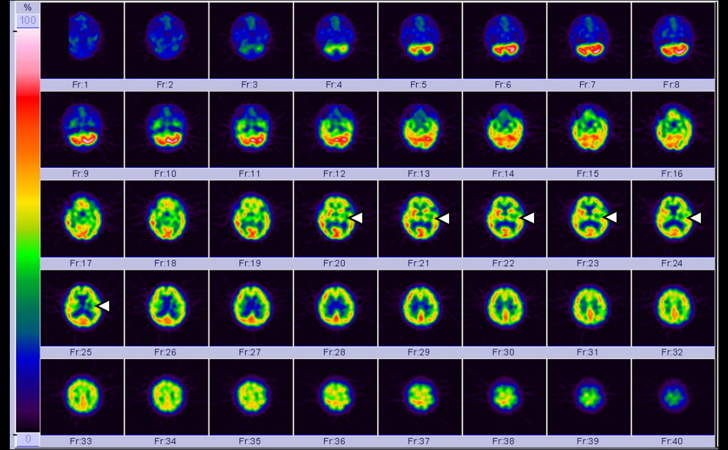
SPECT. SPECT using 99mTc-ethyl cysteinate dimer showed extensive hypoperfusion in the left lentiform nucleus, thalamus, and cerebral cortex. Arrow head: extensive hypoperfusion in the left lentiform nucleus and thalamus. SPECT: Single-photon emission computed tomography.
